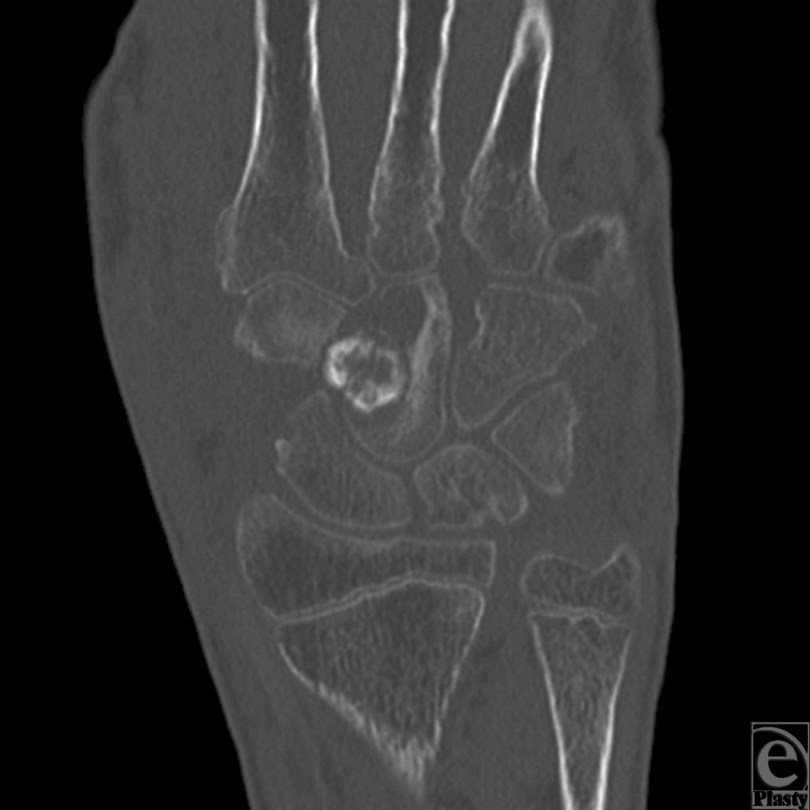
Preoperative computed tomographic scan. The nidus and destruction of the capitate are visible.
